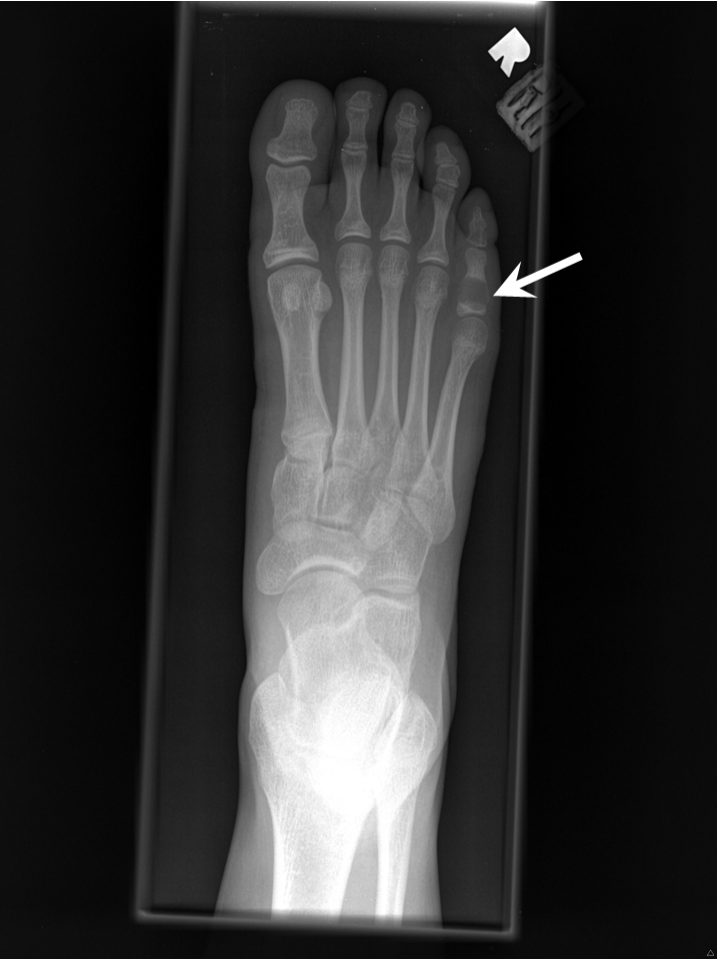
X-ray of the right foot (case no. 1) showing a radiolucent lesion of the proximal metaphysis of the fifth proximal phalanx with scalloped and sclerotic borders, pronounced thinning of the medial and lateral cortices, and without radiographic evidence of an acute fracture, calcification or malignancy (arrow). R indicates the right side.
